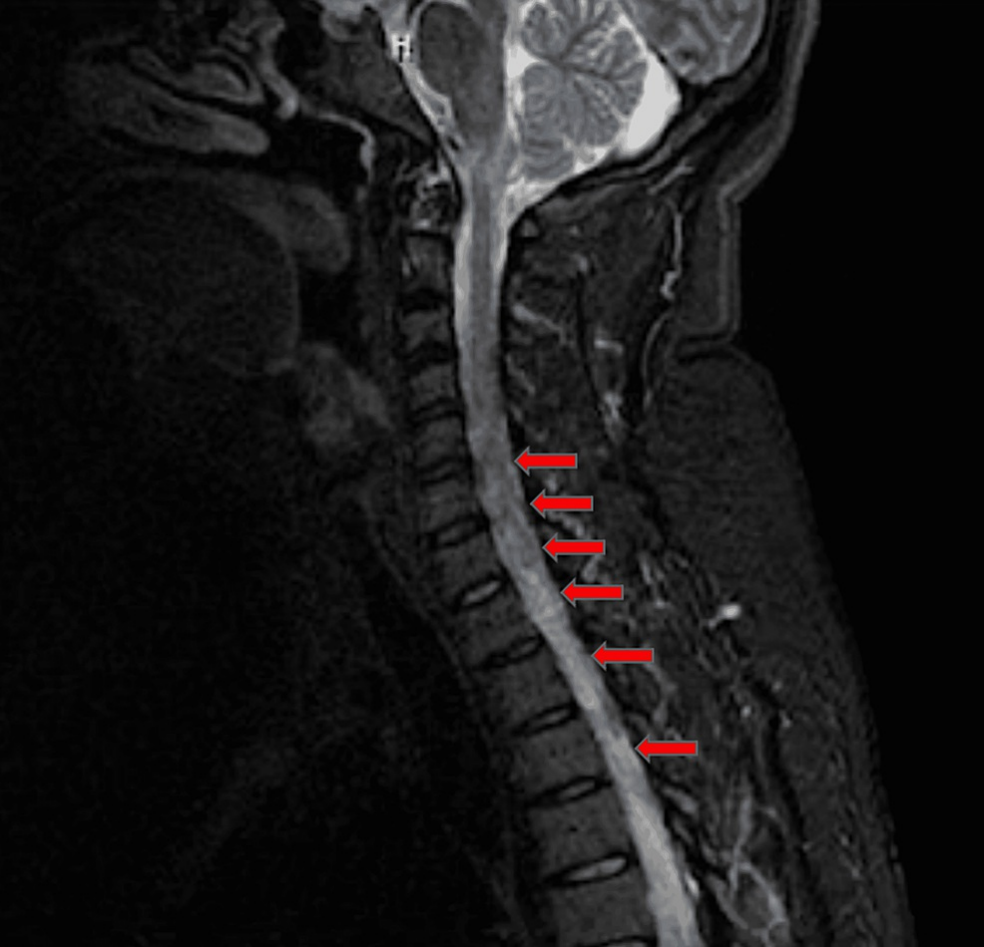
Magnetic resonance imaging of the cervical spine showing signal abnormalities of the cord involving the central aspect with inflammatory etiologies consistent with neuromyelitis optica (red arrows).
